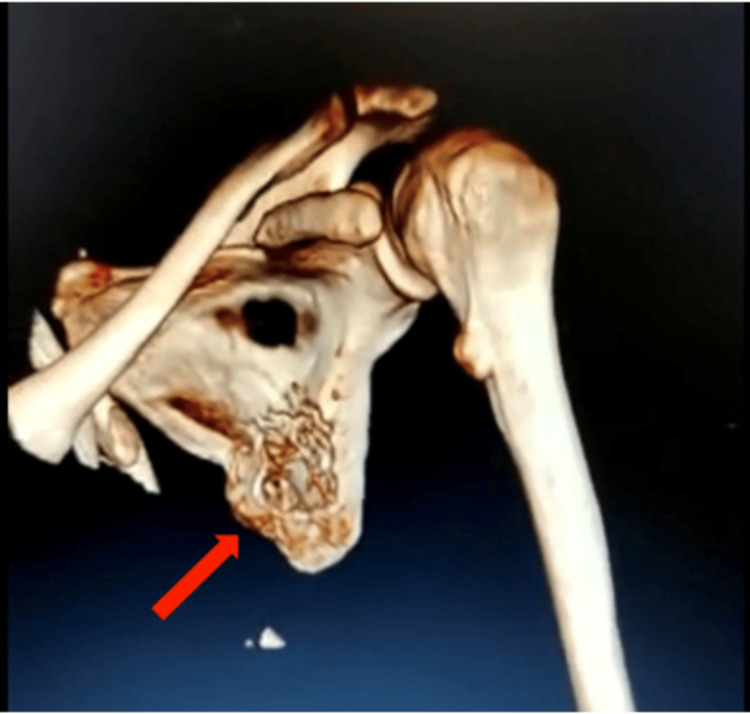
Three-dimensional CT scan showing the excised tumor mass CT: computed tomography
